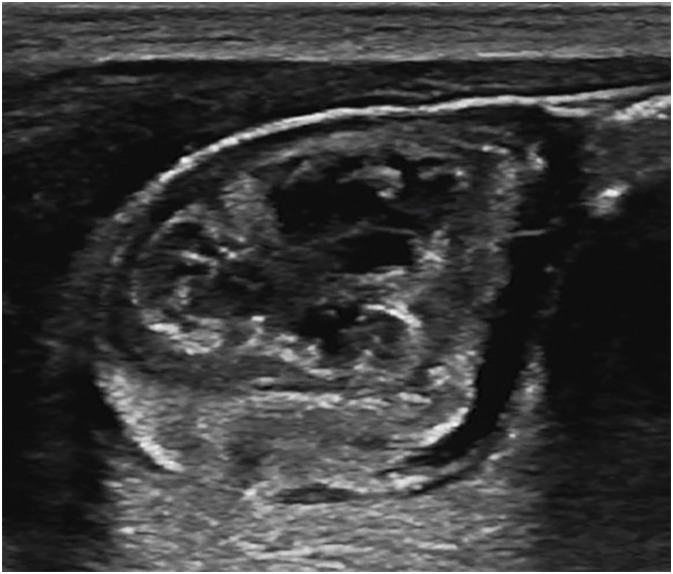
Postnatal ultrasound findings. A 20-mm abdominal mass observed in the right lower abdomen.
